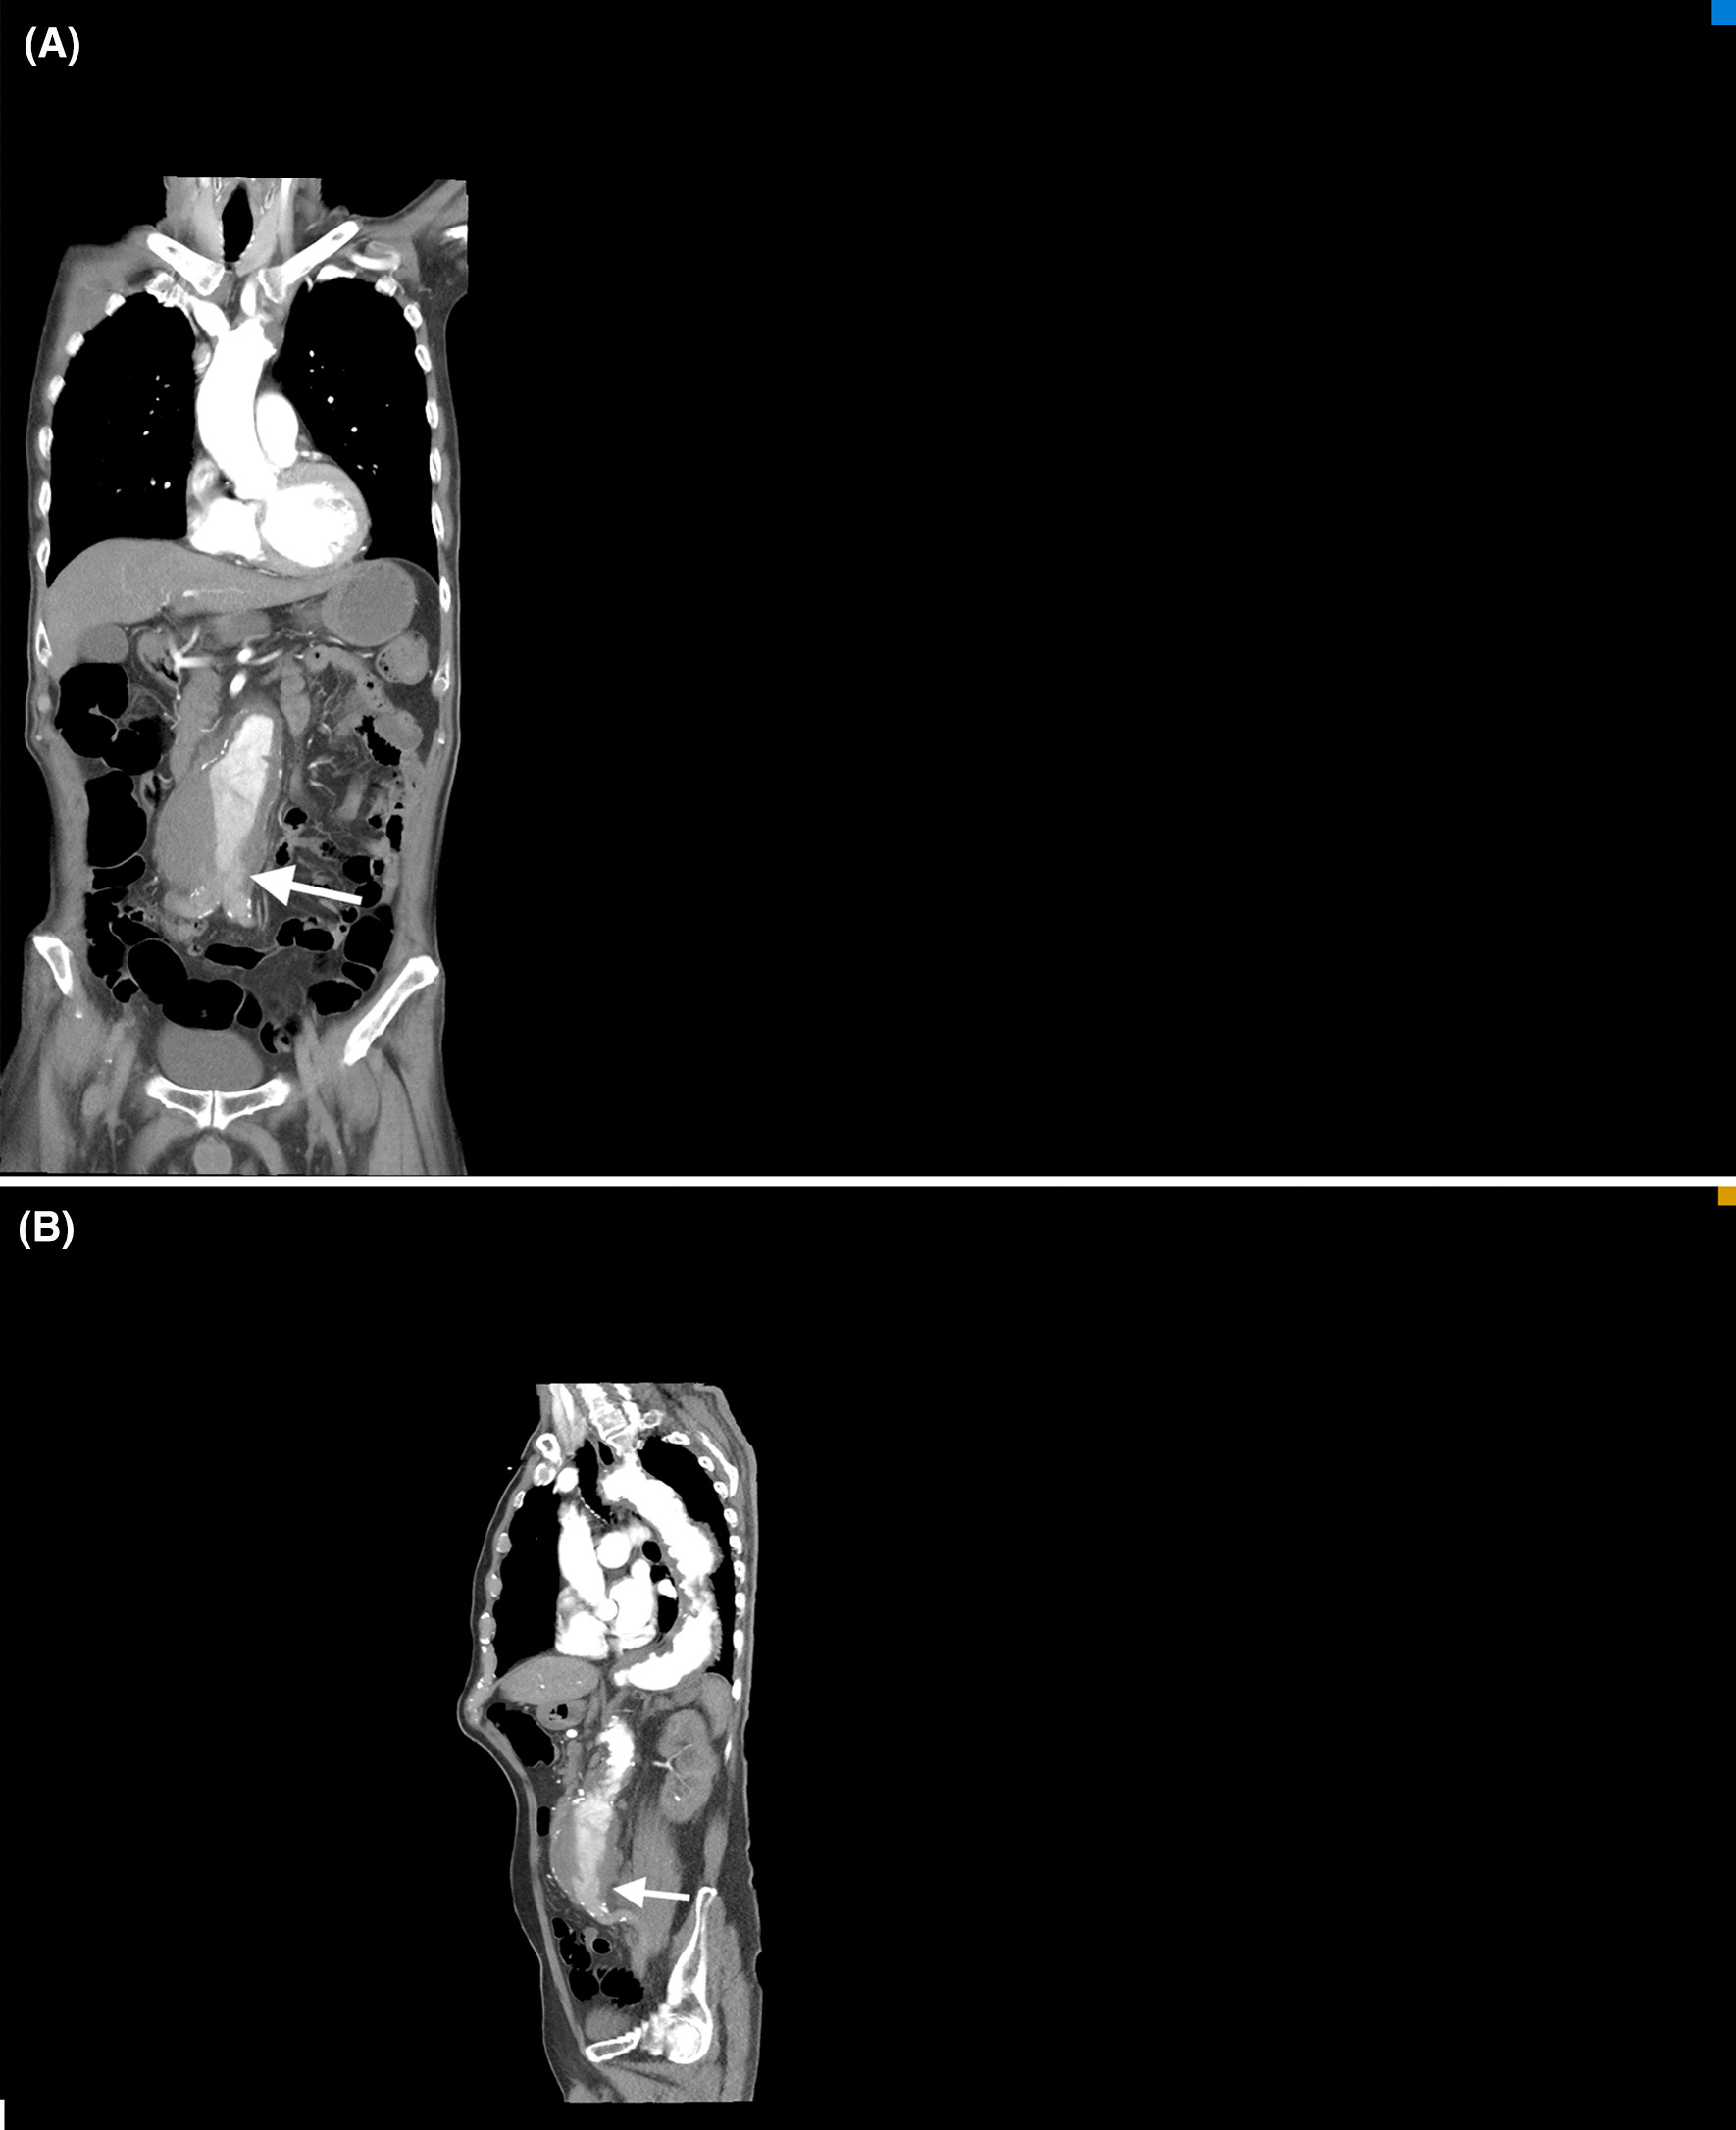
CTA in arterial phase was performed A with no contrast medium in the inferior vena cava in coronal view. There was no signal detection below the aortoiliac bifurcation. B Sagittal view revealed highly irregular mural thrombus lobulated in the aneurysm with focal flap formation related to dislocation of chronic mural thrombus burden (The both white arrows show a floating flap of thrombus near the aortic bifurcation)
